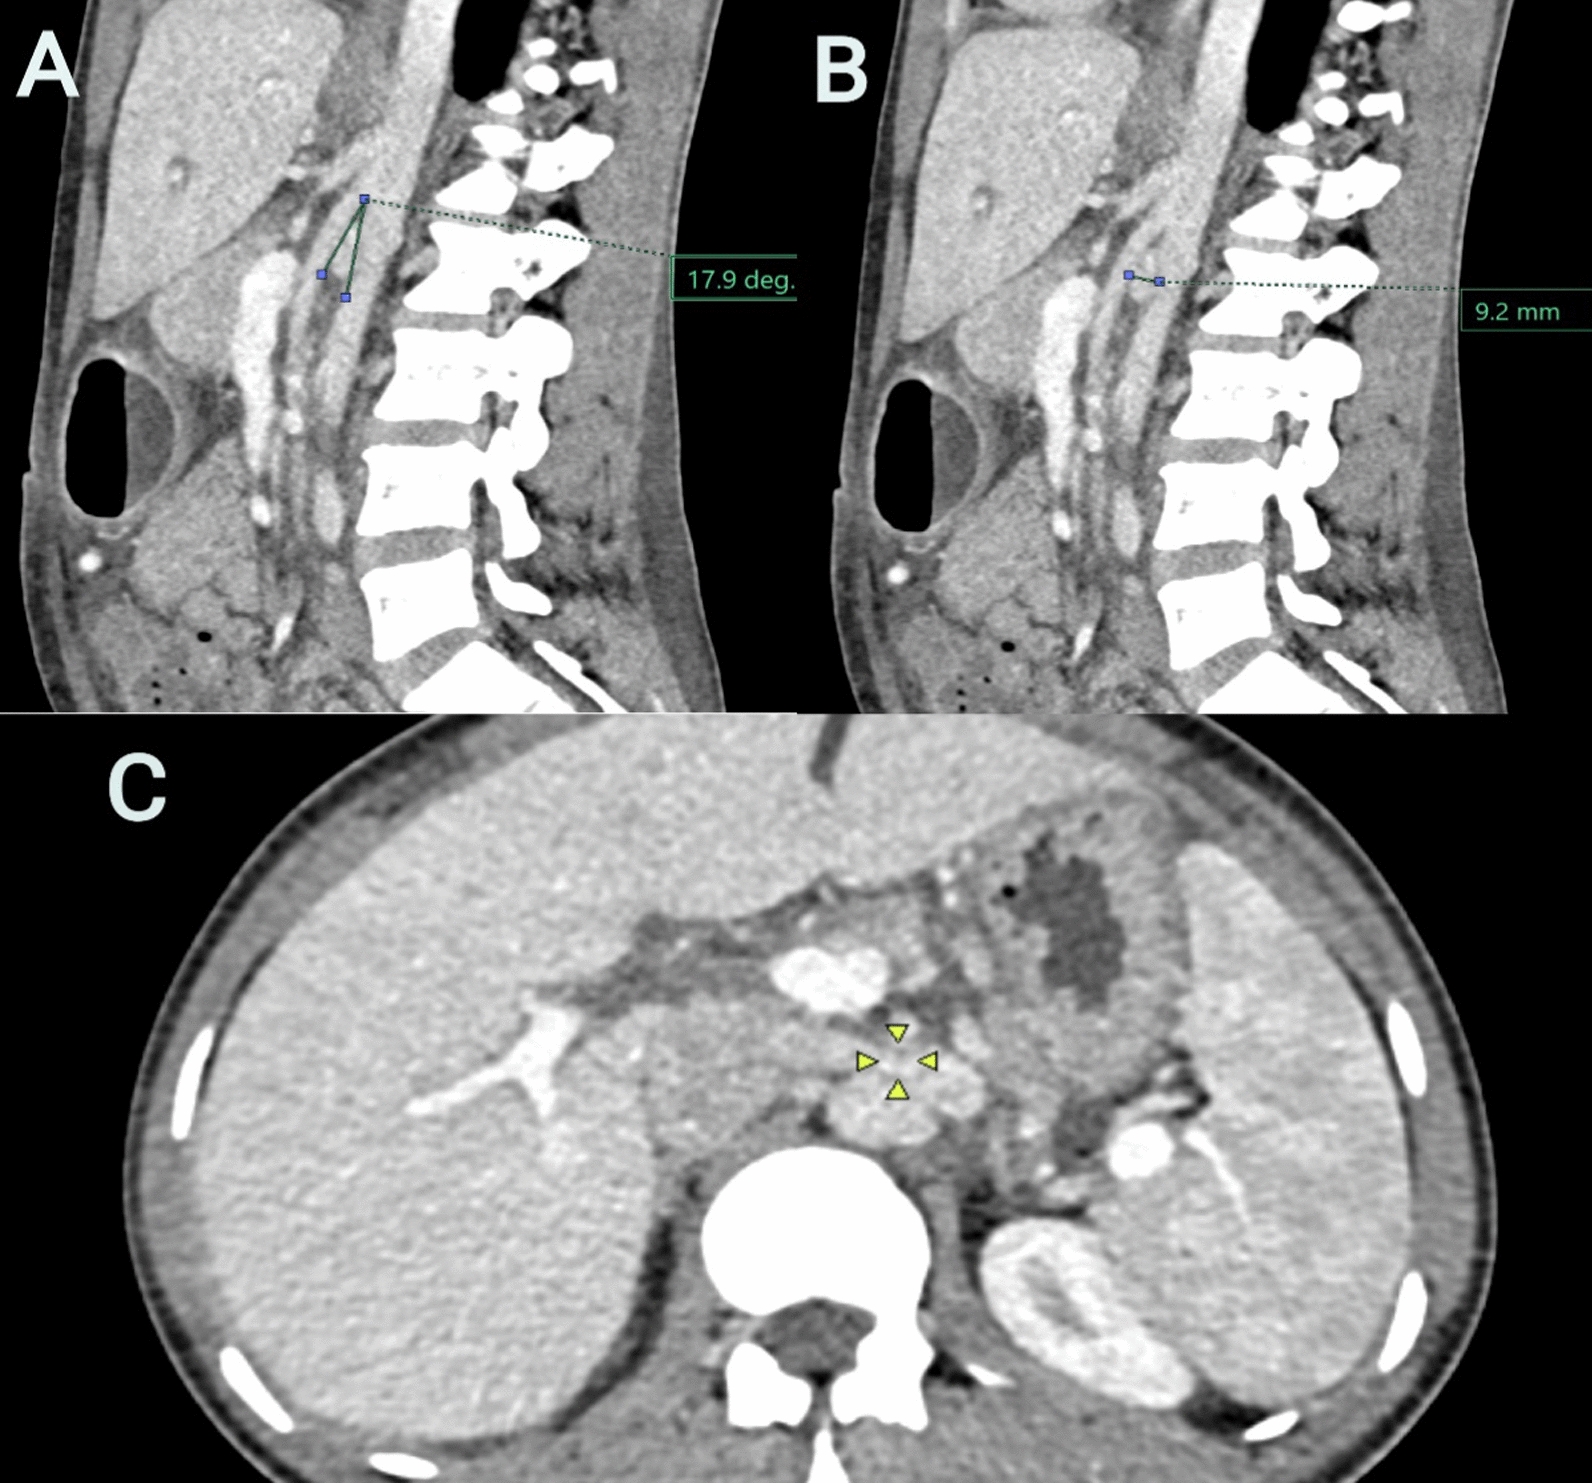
A Computed tomography angiogram sagittal section showing an aortomesenteric angle of 17.9 degrees with minimal dilatation of gonadal vein; B computed tomography angiogram sagittal section showing an aortomesenteric distance of 9.2 mm; C computed tomography angiogram axial section showing dilated renal vein (arrows)
